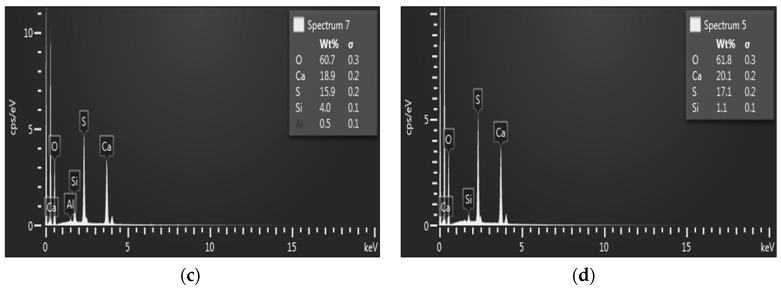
Energy dispersive X-ray microanalysis (EDX) semi-quantitative analysis of (a) M0-6; (b) M1-6; (c) M0-2; and (d) M1-2 samples.
